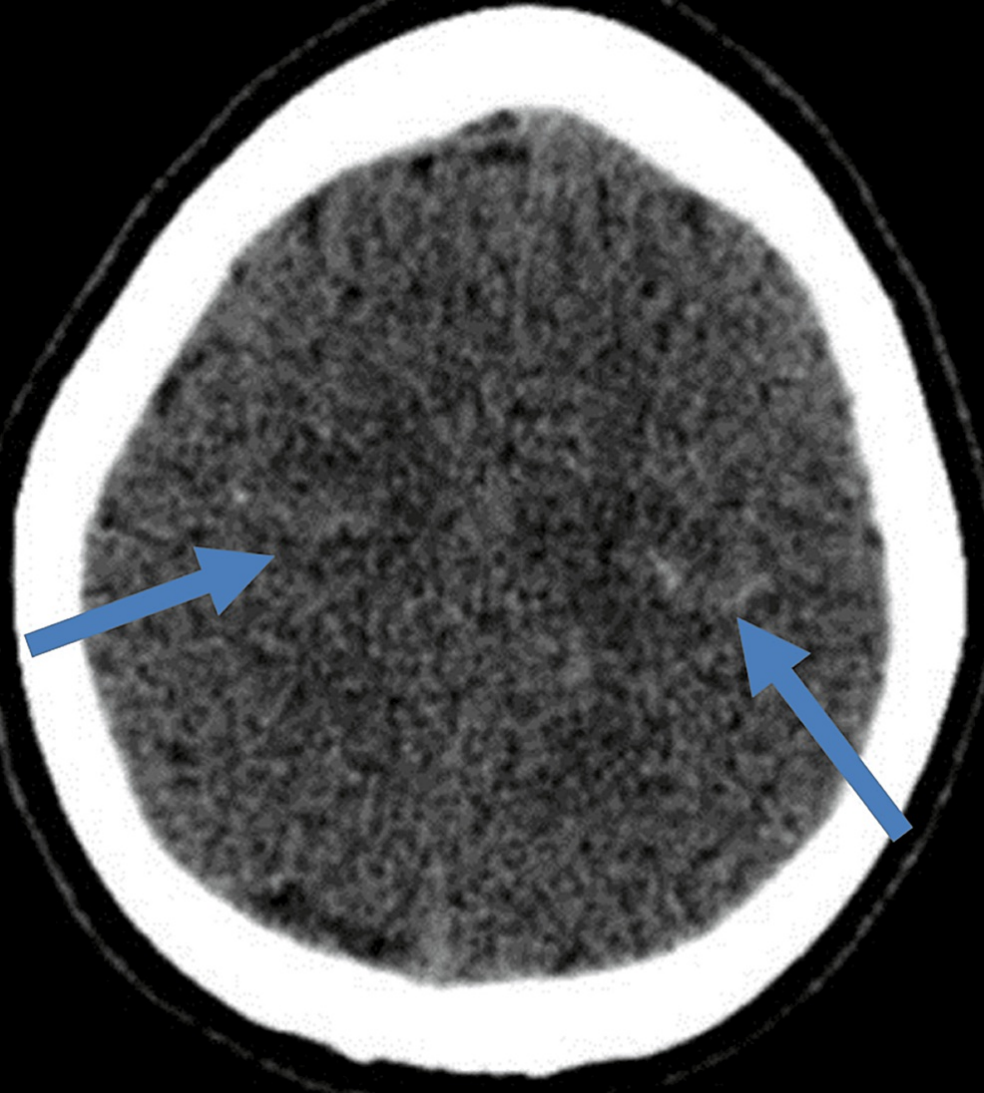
A 60-year-old man with a sudden, severe frontal headache accompanied by vomiting. Unenhanced CT shows focal SAH (blue arrows) in bilateral precentral sulci. CT angiogram (not shown) was normal. CT: computed tomography; SAH: subarachnoid hemorrhage
